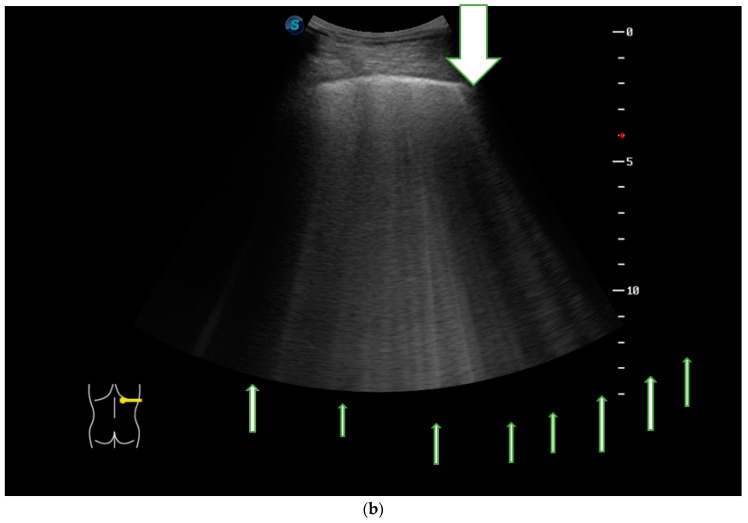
(a) HRCT imaging in the lung window at the level of middle and lower lobes with subpleural honey combing and fine reticulation, as well as traction bronchiectasis, that confirm UIP pattern (black lines). (b) LUS imaging with multiple B-lines (thin arrow), confirm the fibrotic interstitial syndrome; thick arrow represents pleural line which is not thickened.
